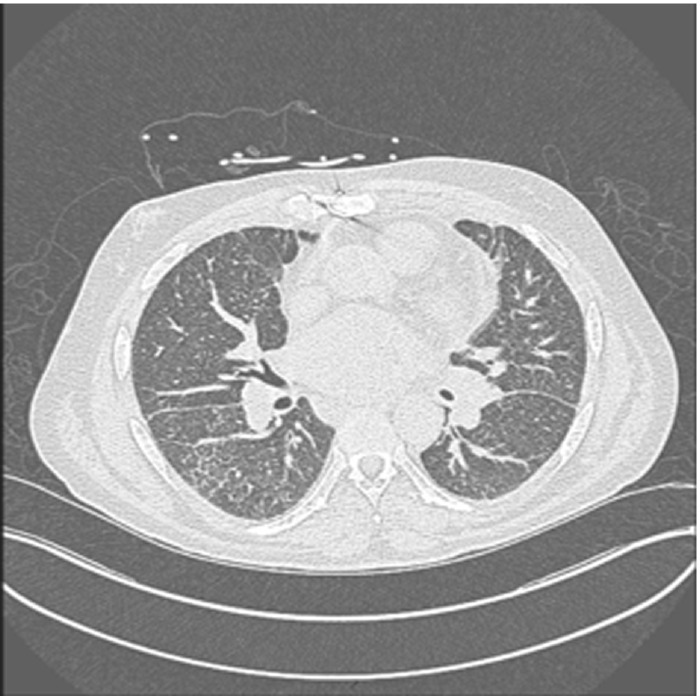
Initial CT scan revealing hilar adenopathy, LUL consolidation versus mass and chronic nodular septal thickening
